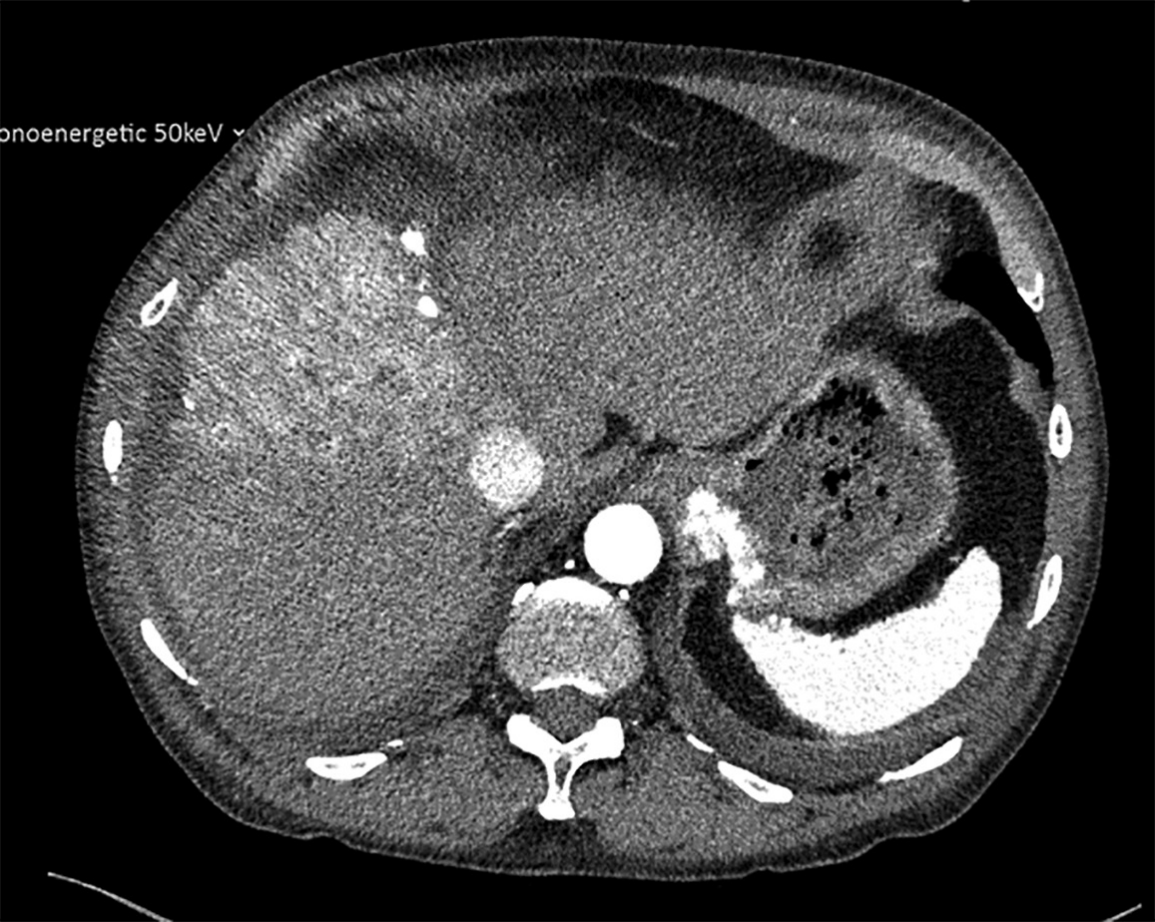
Computed tomography of the upper abdomen at the time of referral to our department: hypervascularized tumor focus in segment VIII/IVa (9 × 9 cm) with washout in the late phase and necrotic arreals.
